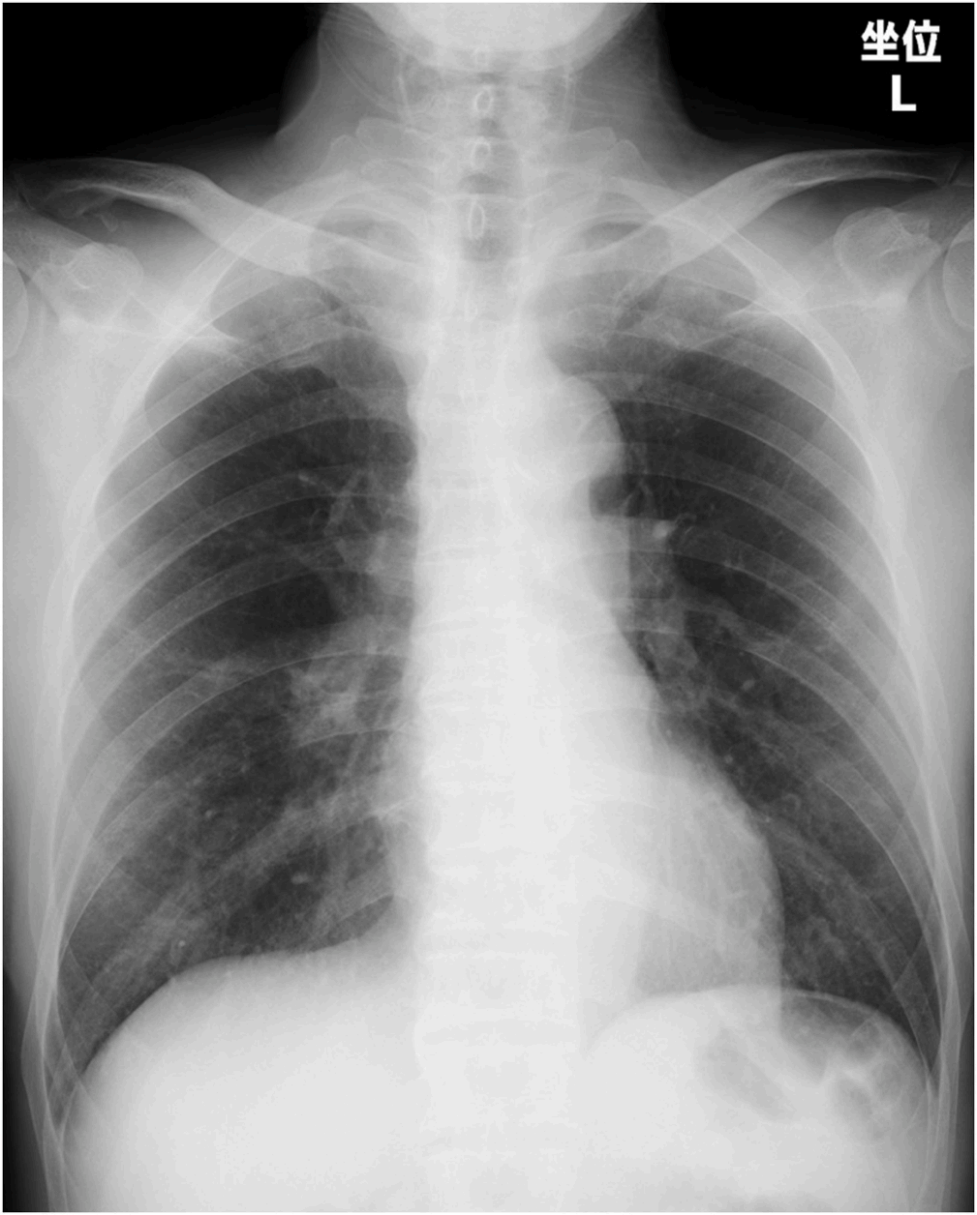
Chest radiograph of the initial visit showed no significant abnormalities.
